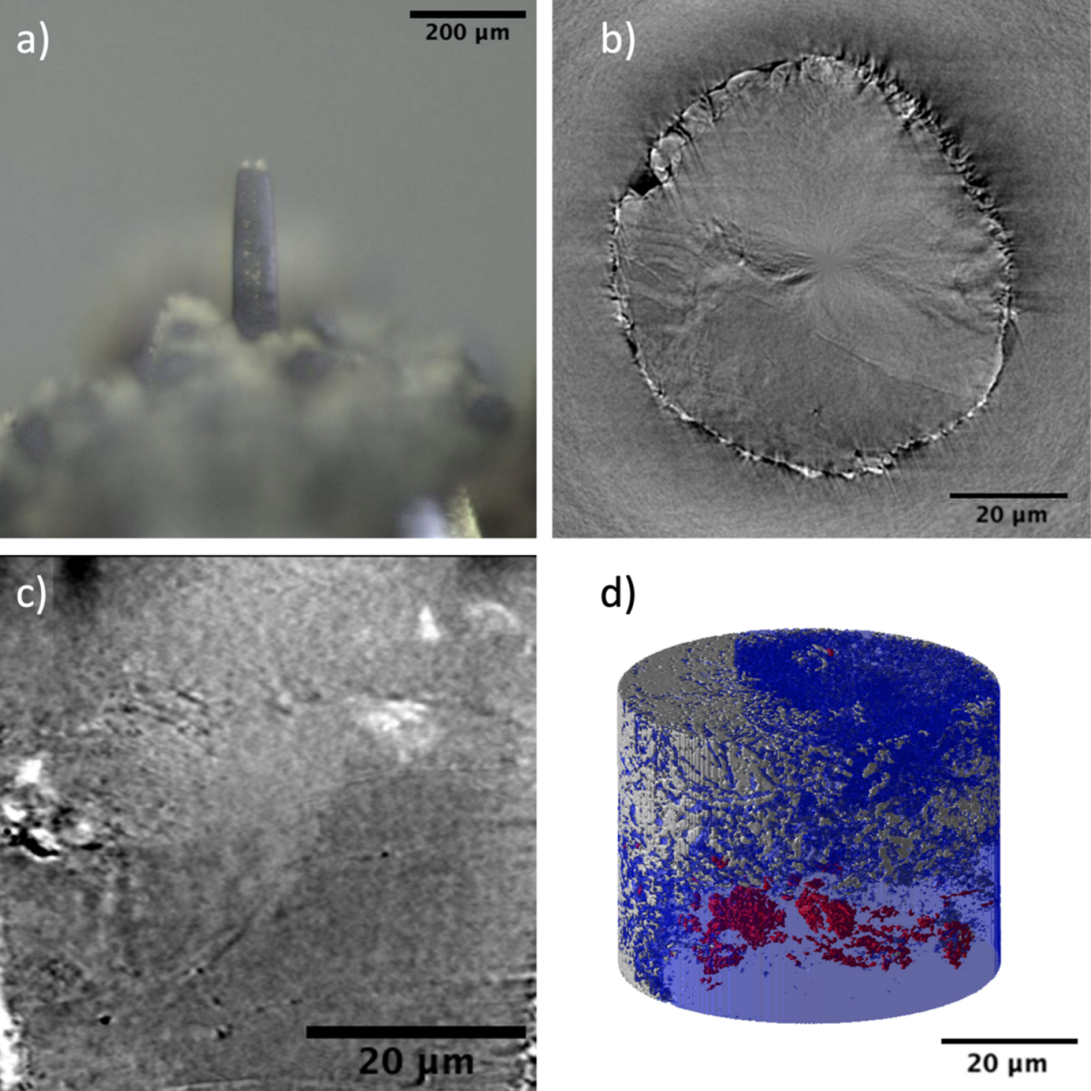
(*a*) Optical image of the serpentinite pillar sample for nano-CT. (*b*) Horizontal and (*c*) vertical slice of the reconstructed nano-CT data. Also, (*d*) 3D visualization of the three mineral phases in the sample with gray, blue and red representing dark gray, light gray and bright mineral phases, respectively.
